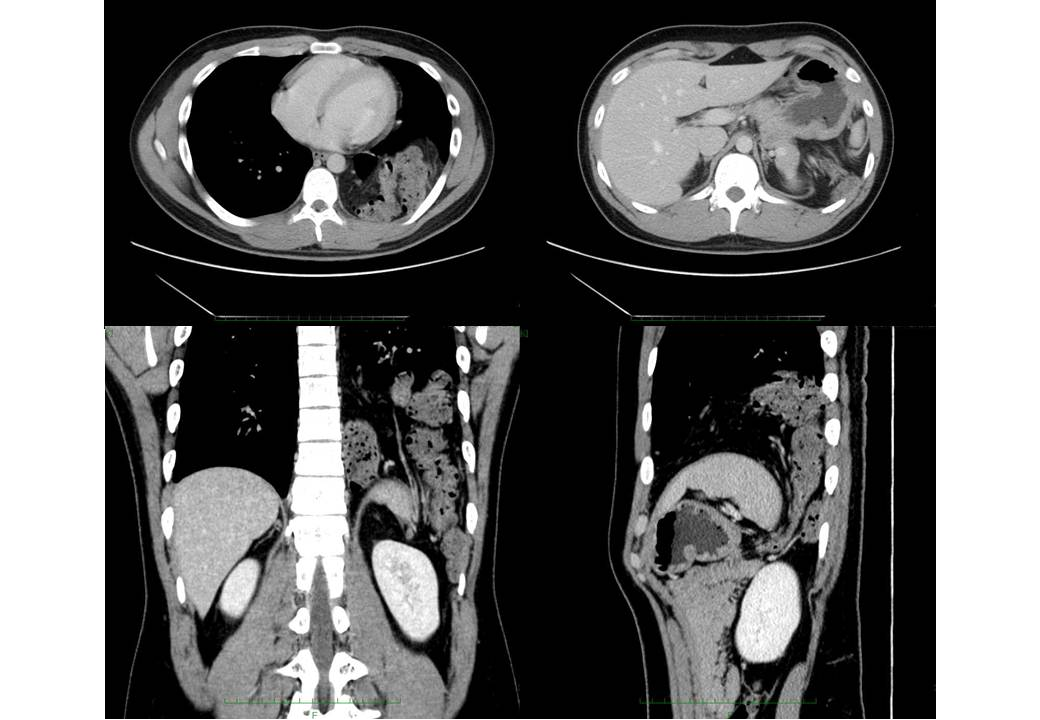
Preoperative enhanced chest and abdominal computed tomography (CT) scans. The chest CT shows a diaphragmatic hernia. The spleen and left colon have herniated into the left thoracic space through a left posterior diaphragmatic defect.
